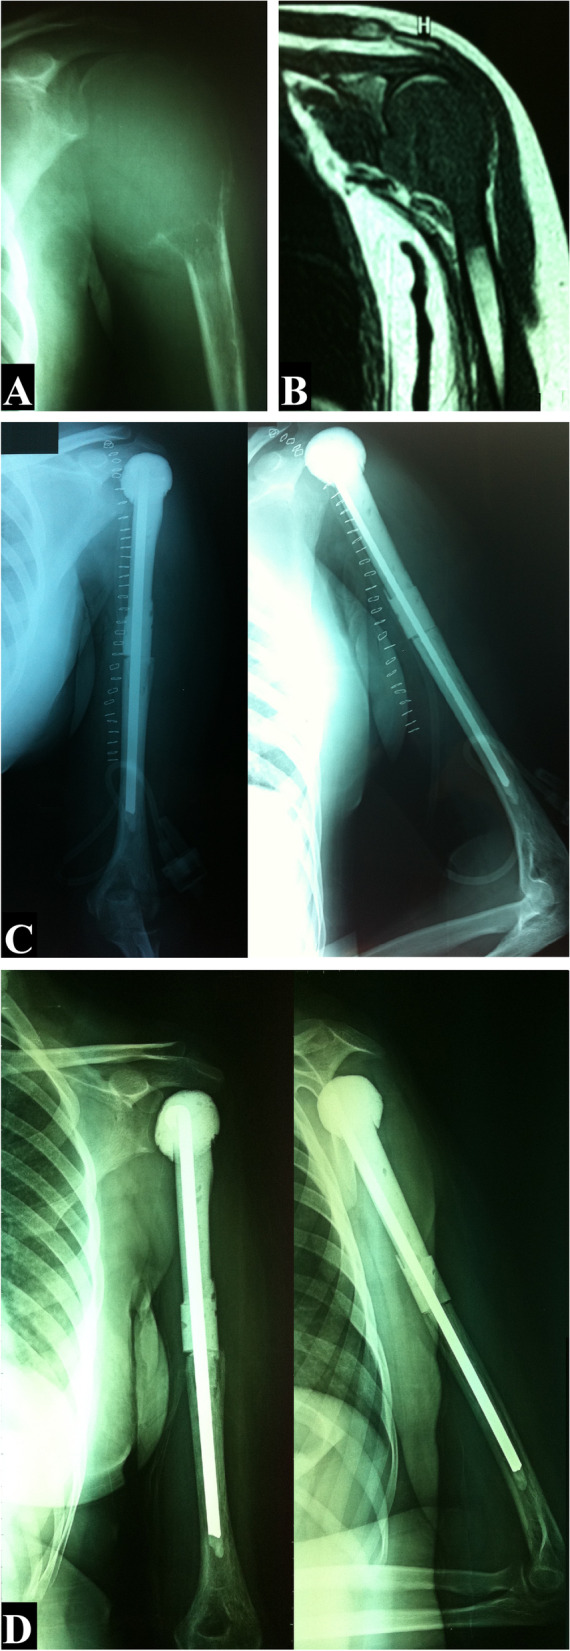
A 22-year-old female patient with giant cell tumor of the proximal humerus had undergone tumor resection and reconstruction with cement spacer. A Plain X-ray of the shoulder, anteroposterior view. B MRI sagittal view. C Postoperative X-rays, anteroposterior and lateral views. D Two-year follow-up X-rays, anteroposterior and lateral views
